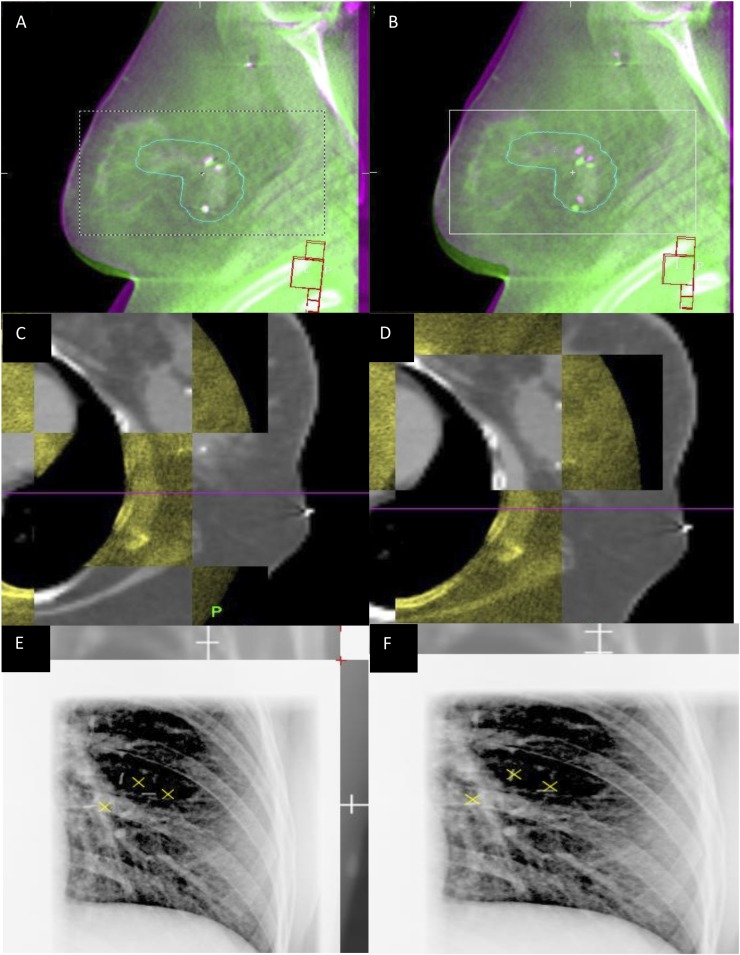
Example images used for setup error analysis. (a) and (b) show sagittal planning CT images (pink) overlaid with sagittal cone-beam CT images (green). Figures (c) and (d) show planning axial CT images in grey and axial megavoltage CT in yellow. Figures (e) and (f) show two-dimensional kilovoltage projection images overlaid on digitally reconstructed images from the planning CT; planning CT clips positions are marked with yellow crosses. Images in the left-hand column (a, c and e) show bone-matched images and images in the right hand column (b, d and f) show clip-matched images. For colour image see online.
